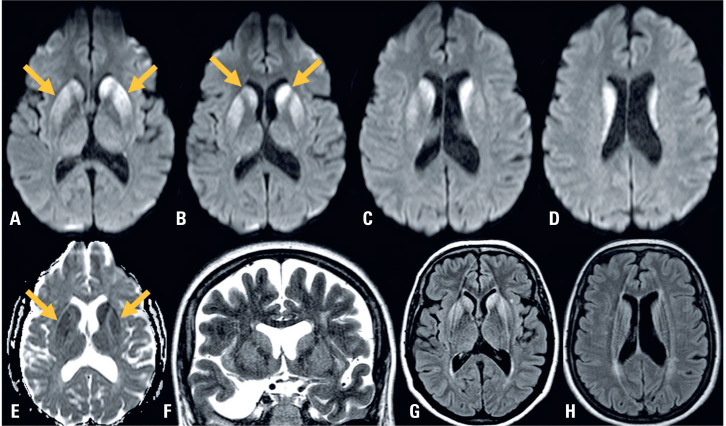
Axial diffusion-weighted imaging (A–D) showing hyperintensity involving the bilateral putamen (yellow arrows) and caudate heads (yellow arrows), with hypointensity on the apparent diffusion coefficient map (yellow arrows in E), hyperintensity on coronal T2-weighted imaging (F), and axial T2 fluid-attenuated inversion recovery (FLAIR, G, H). No cortical changes were observed on any of the MRI sequences
